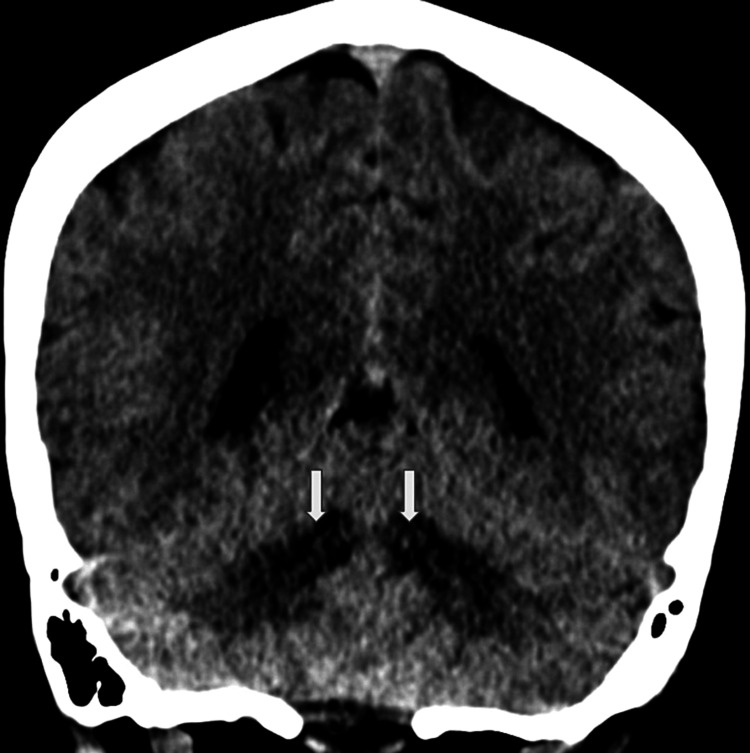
CT coronal reconstruction demonstrating abnormal symmetric cerebellar low densities (indicated by arrows).
